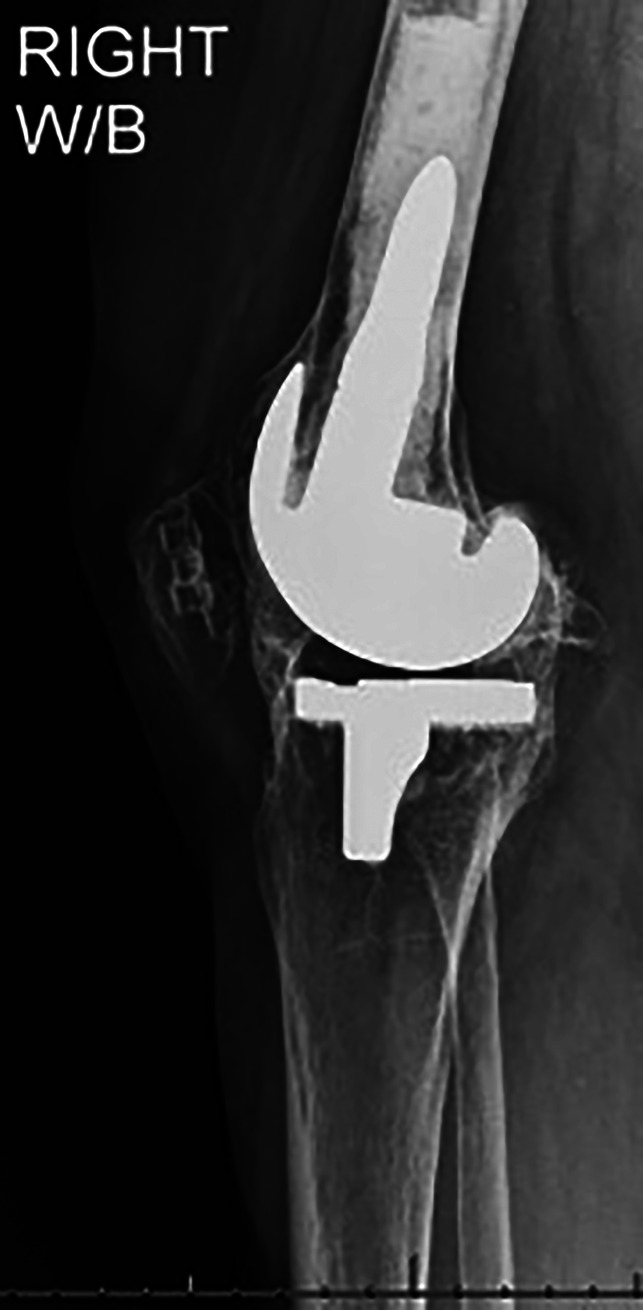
Lateral radiograph of the right knee.
